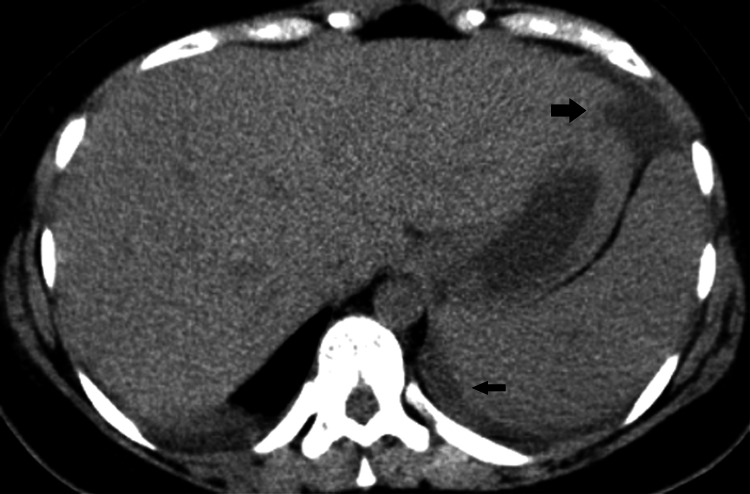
Axial non-enhanced computed tomography image Low-density fluid (arrow) adjacent to the spleen due to subcapsular hematoma. There is free fluid in the greater sac (thick arrow).
